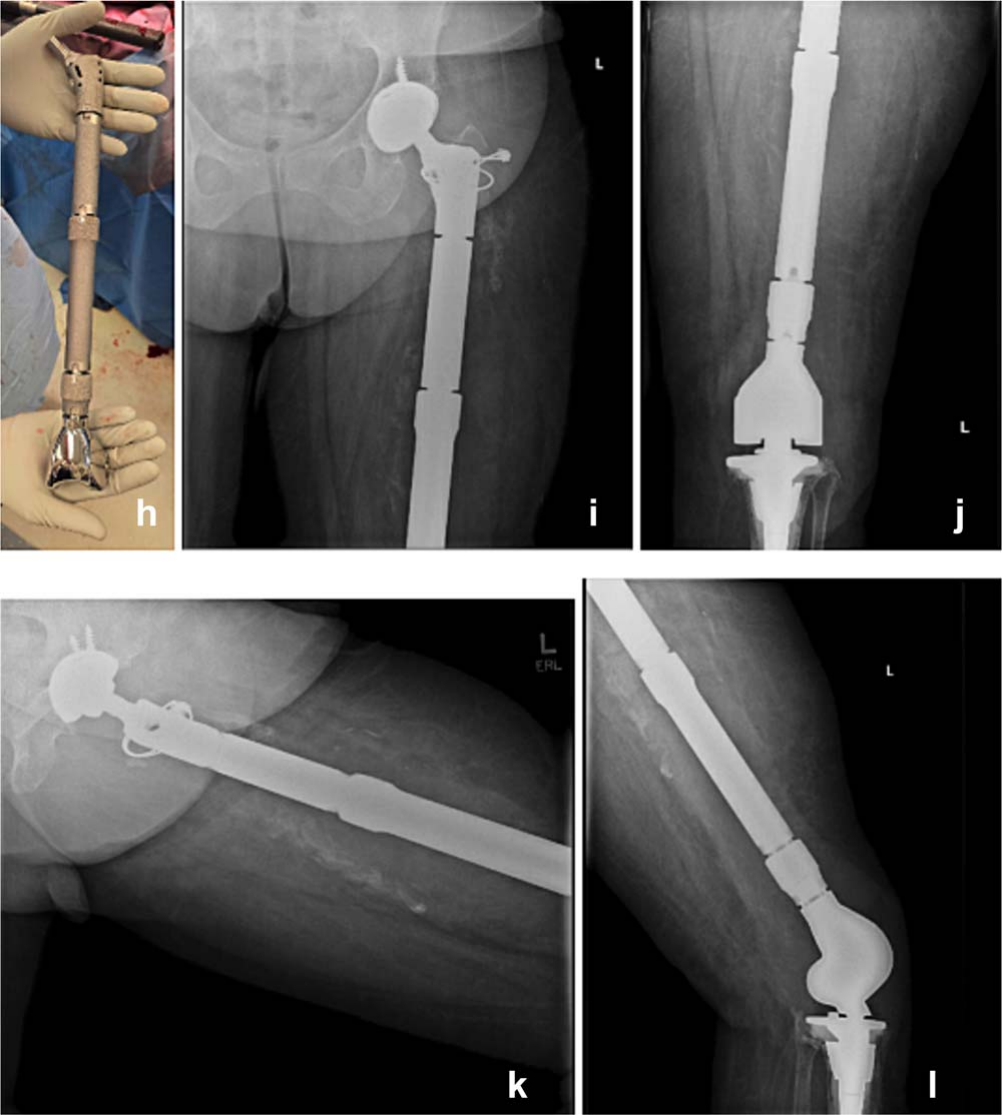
The modular TFR implant (h) is grossly visualized prior to implantation. Immediate postoperative anteroposterior (i and j) and lateral (k and l) radiograph following definitive reimplantation, showing the modular total femur replacement with a dual mobility acetabular component proximally and a rotating-hinge knee mechanism distally in appropriate alignment.
